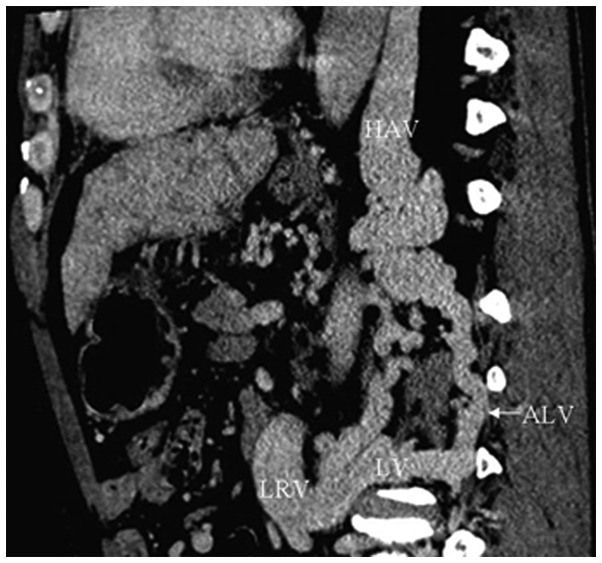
IVC-left renal vein-ALV-hemiazygos vein collaterals. Computed tomography angiography shows the connection of the dilated left renal vein with the apparently dilated and tortuous HAV by means of the LV and ALV. IVC, inferior vena cava; LV, lumbar vein; ALV, ascending LV; HAV, hemiazygos vein.
